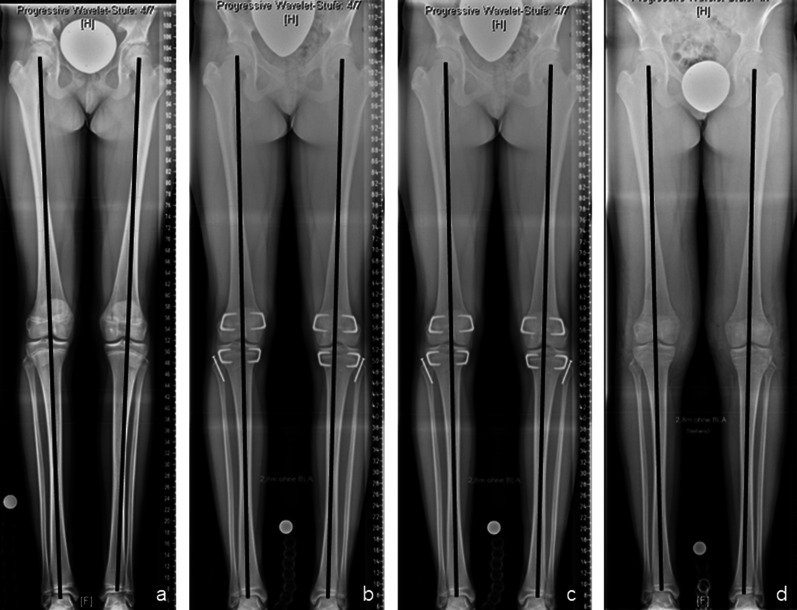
Results of bilateral temporary epiphysiodesis using rigid staples (RigidTacks™) around the knee in a girl with idiopathic (familial) tall stature. Anteroposterior long standing radiographs right before surgery at the age of 12 years (a), after 1 year (b) and 2 years (c) of temporary growth arrest, and right after hardware removal at skeletal maturity (d), showing physiological coronal alignment of the knees and equal leg lengths during and after treatment
